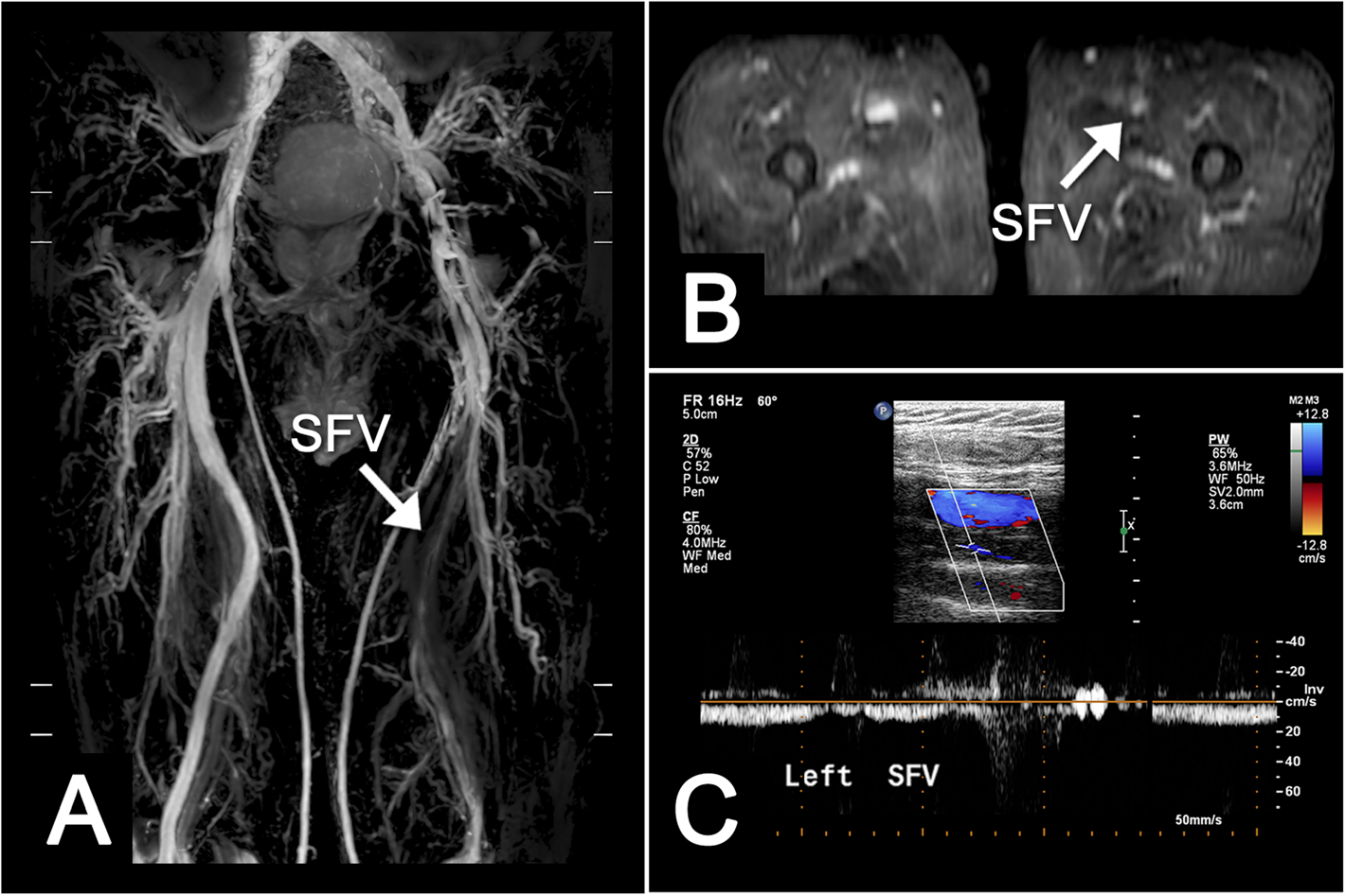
Deep vein thrombosis (DVT), Coronal (a) and axial (b) images showed incomplete opacification of left superficial femoral vein (SFV), consistent with DVT. Doppler ultrasound also showed the same result (c)
